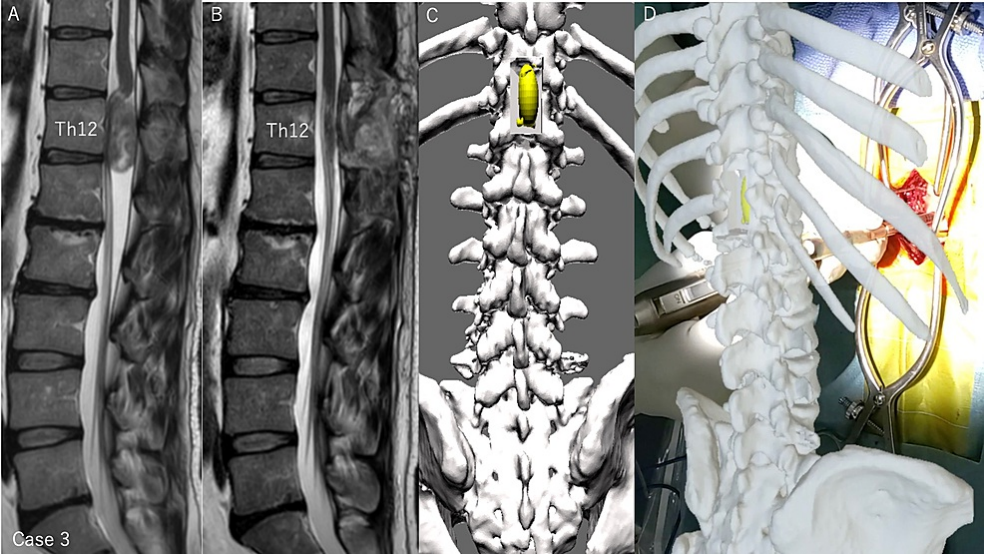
Pre- and postoperative MRI and 3D model of thoracic tumor case and intraoperative support with 3D model (case 3) (A) Preoperative MRI T2-weighted sagittal section showed a tumor including a cyst at the posterior part of Th12 vertebral body. (B) Postoperative MRI T2-weighted sagittal section showed no residual tumor and the laminectomy of Th11 caudal and Th12 lamina. (C) Dorsal view of the post-laminectomy model, in which the Th11 caudal and Th12 lamina were removed with a width of 20 mm. The margins of the spinal cord tumor can be seen, confirming that the tumor can be safely removed with this laminectomy. (D) Intraoperatively, the HMD could be used to confirm that the laminectomy was performed as planned in the surgical field.
